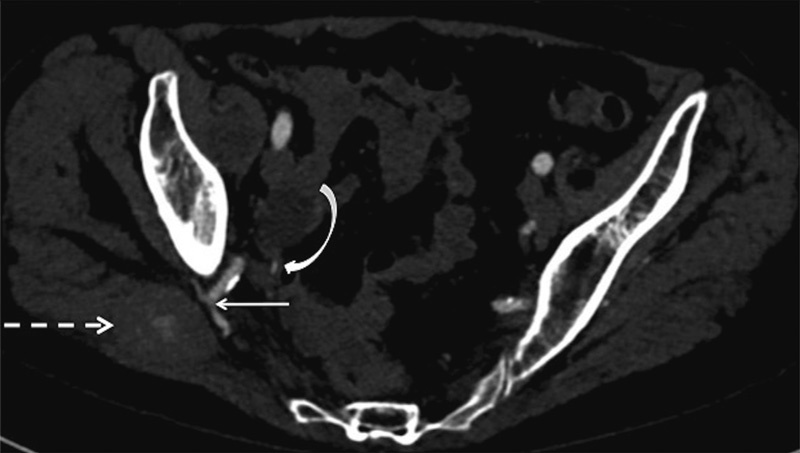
Axial computed tomography angiogram demonstrates enhancement of the superior gluteal artery (straight white solid arrow), which is adjacent to a fusiform mass (dotted white arrow) within the gluteal muscles. The mass demonstrates internal contrast blush in keeping with a pseudoaneurysm. The curved white arrow indicates the inferior gluteal artery.
